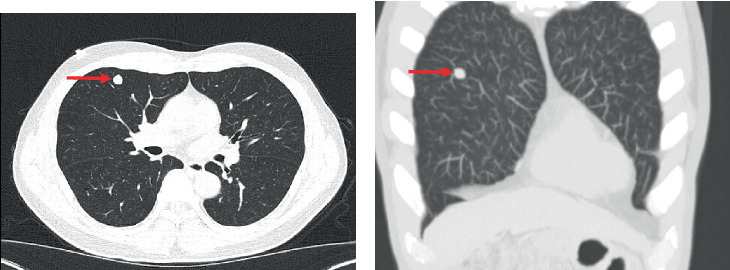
Low-dose noncontrast CT (lung cancer screening protocol) showing a 1-cm right upper lobe pulmonary nodule in both (a) axial view and (b) coronal view.
